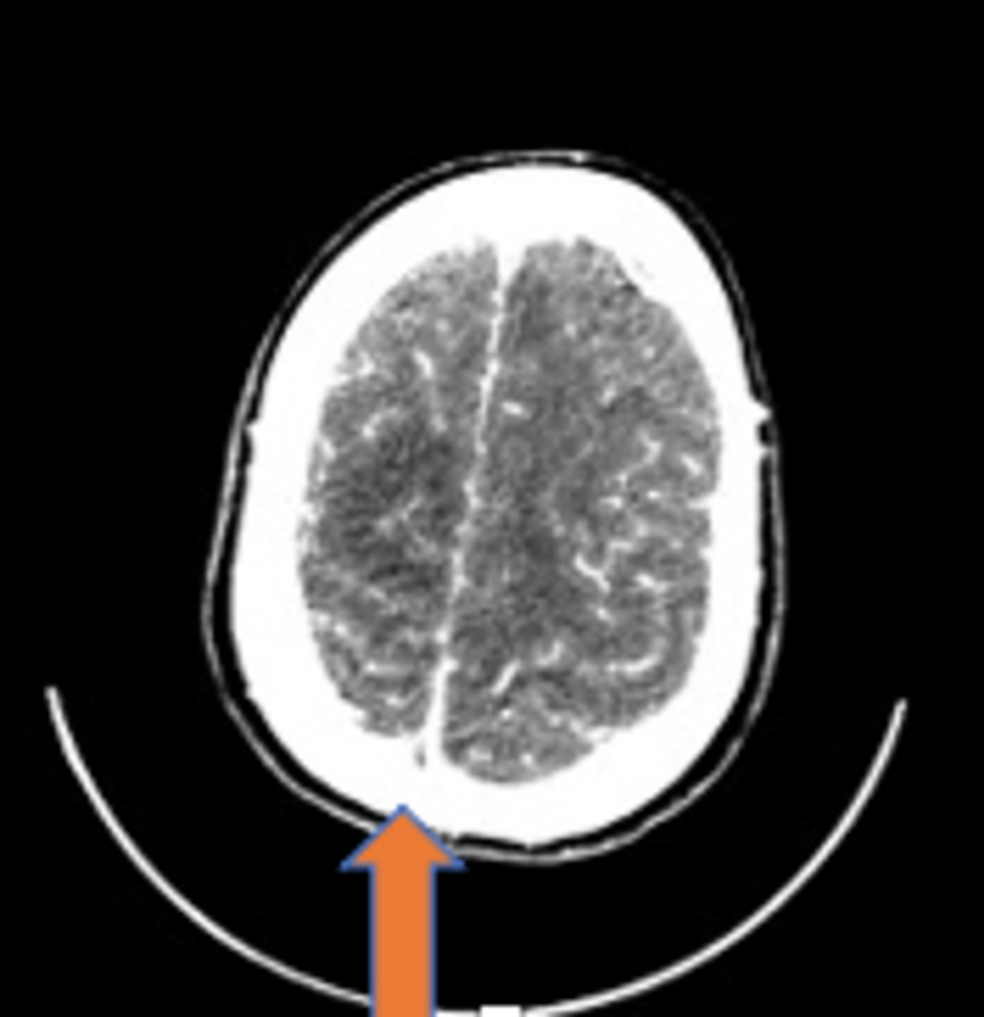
CT with contrast showing extensive superior sagittal sinus thrombosis
